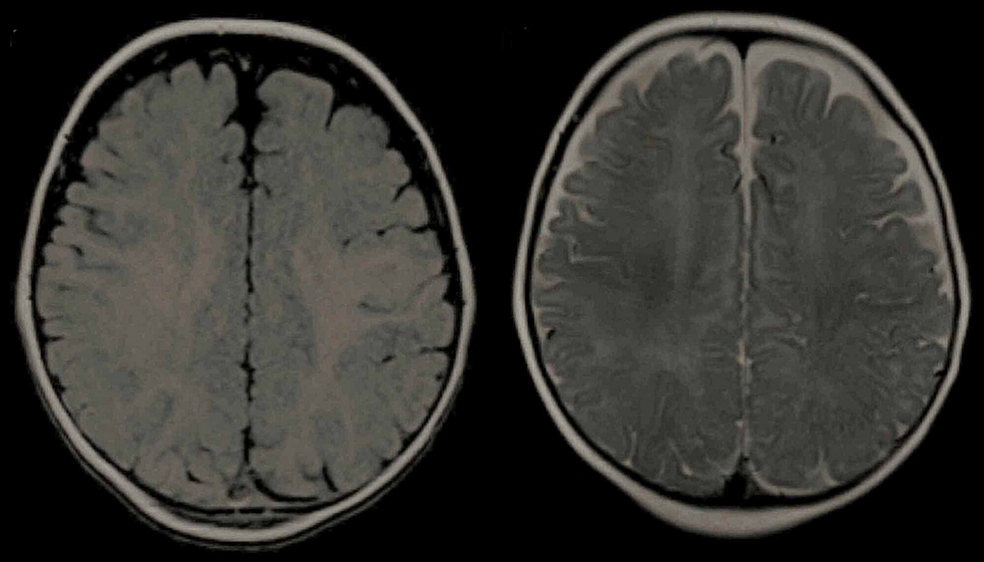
MRI in T1 and T2 sequences MRI: magnetic resonance imaging
